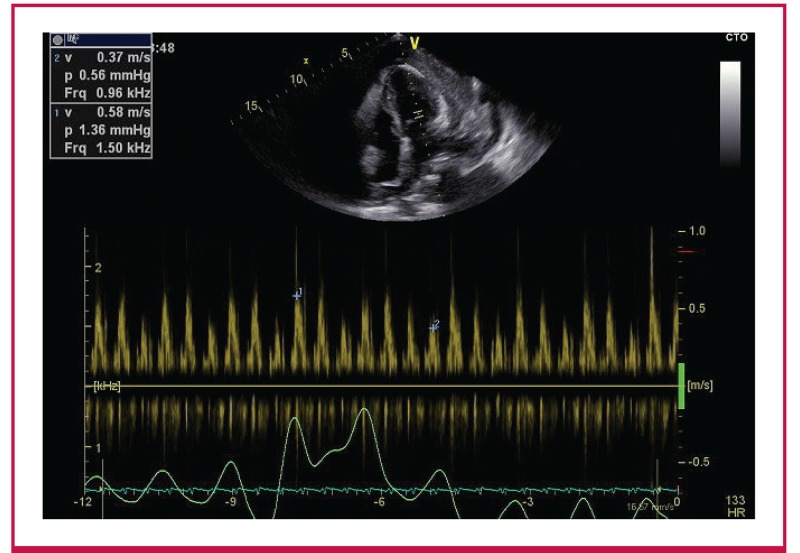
Pulse-wave Doppler at the level of the mitral valve leaflet tips demonstrating a respiratophasic variation in the early diastolic transmitral inflow velocities in excess of 25%.
